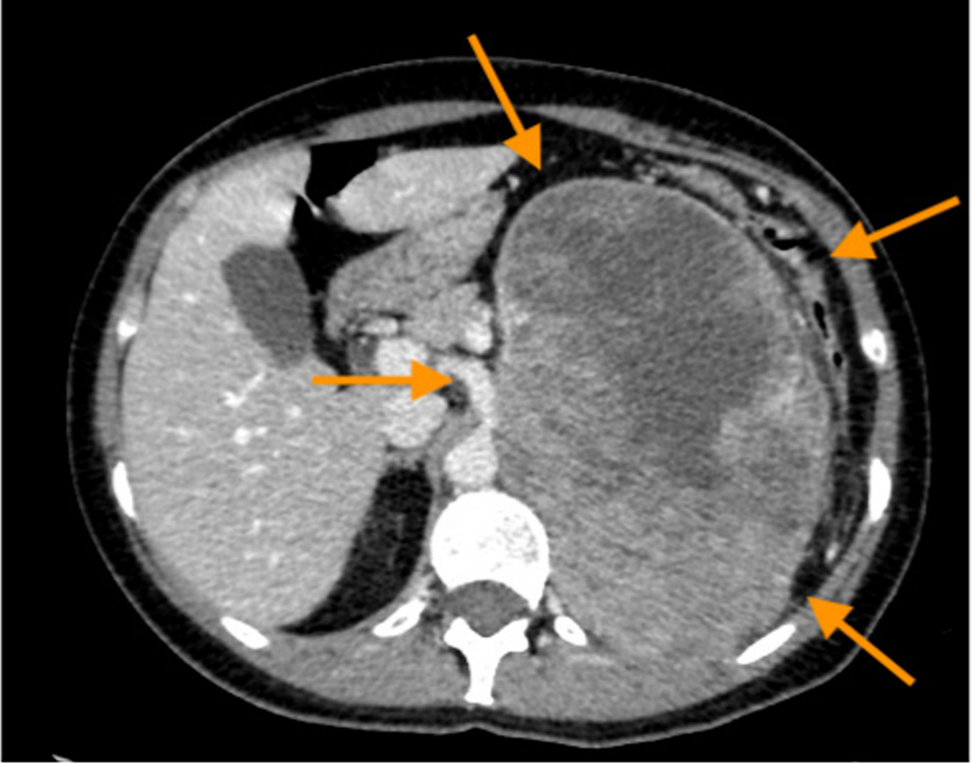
CT-scan, the imaging revealed a heterogeneous retroperitoneal mass of 17 cm in diameter containing necrotic and hemorrhagic areas, at the level of the superior left renal pole infiltrating partially the diaphragm (arrows).
